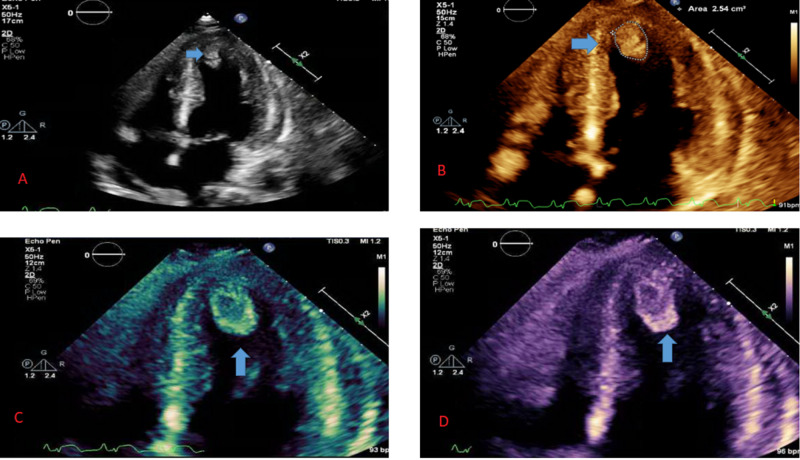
Echocardiogram with apical four-chamber view showing thrombus in the left ventricle, which was likely the source of embolus causing acute limb ischemia (panel A showing apical four-chamber view in gray-scale two-dimensional image and panels B-D showing colored images of the apical view for better visualization of the thrombus which is shown by blue arrows).
